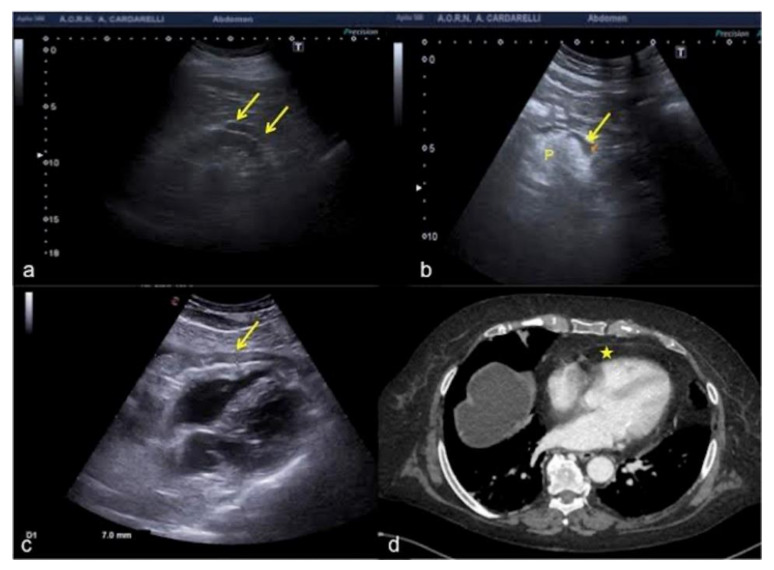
(a) Hypoechoic peri-renal fat, (b) peri-pancreatic fat and (c) pericardial fat misinterpreted as fluid collections (arrows). (d) On CT scan pericardial fat was clearly visible without any fluid collection (star) unlike what was wrongly diagnosed on US scan (c). P: pancreas.
